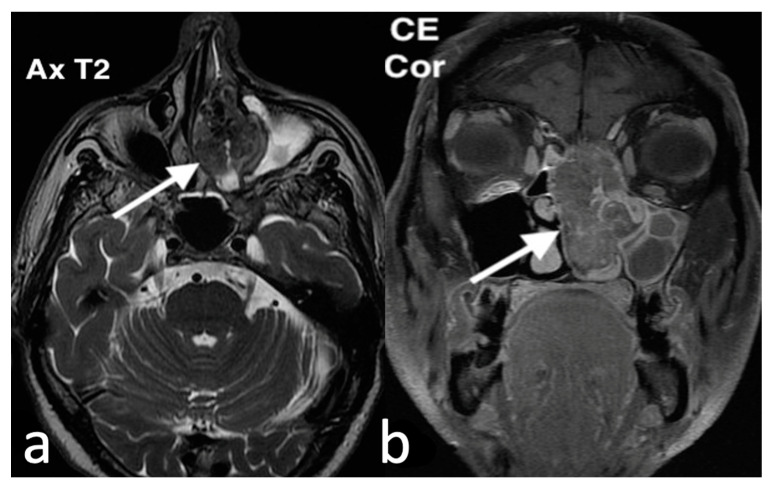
Sinonasal intestinal-type adenocarcinoma in a patient with left nasal obstruction, epistaxis and headache. During rhinoscopy, an irregular-surfaced mass with high tendency to bleed was observed. MRI: the axial T2 image (a) shows a lobulated mass (arrow), in the left nasal cavity, obstructed, which expands the contiguous bony structure and medially erodes the nasal septum, invading the contralateral right nasal cavity; the mass causes obstruction of the ostiomeatal complex; mucocele of the left maxillary antrum is also visible; the coronal T1 after intravenous Gd image (b) shows heterogenous enhancement of the mass (arrow).
